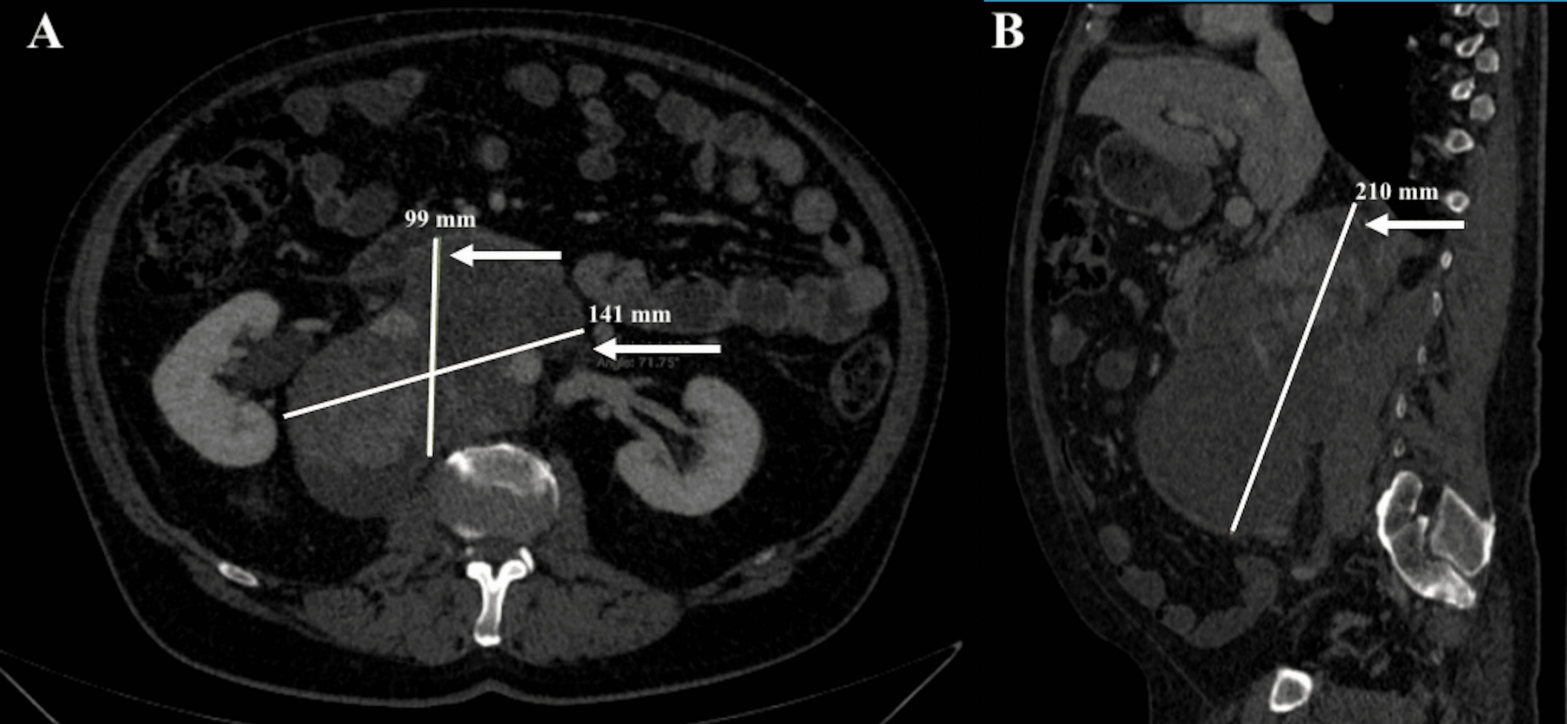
Abdominal and pelvic CT scan at the first follow-up: axial (A) and sagittal (B) planes The image shows a 6.2% reduction in the volume of the retroperitoneal lesion, currently measuring 210 × 141 × 99 mm in the longitudinal (B), transverse, and anteroposterior axes (A), respectively (delineated within the white lines), compared to the previous measurement of 224 × 162 × 111 mm CT: computed tomography
